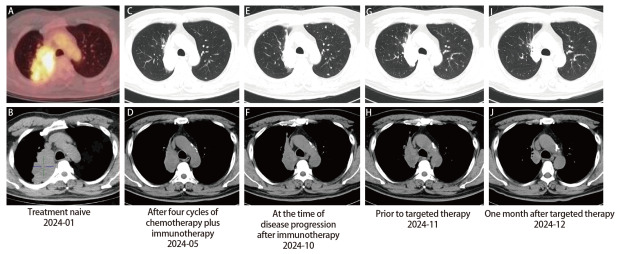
患者病程中胸部影像学检查。 A、B：治疗前（A：PET/CT，B：胸部CT纵隔窗）；C、D：化疗联合免疫治疗4个周期后（C：肺窗，D：纵隔窗）；E、F：免疫治疗后进展时（E：肺窗，F：纵隔窗）；G、H：靶向治疗前（G：肺窗，H：纵隔窗）；I、J：靶向治疗1个月后（I：肺窗，J：纵隔窗）。
